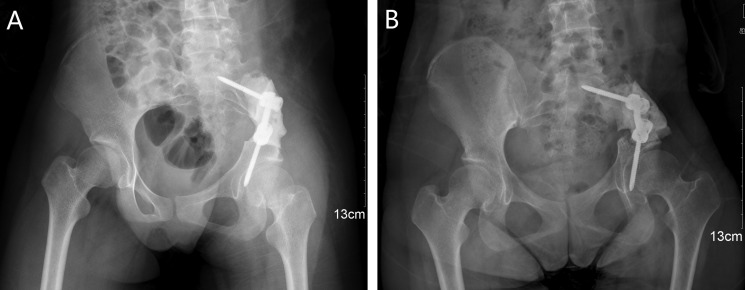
A 15-year-old female (case 28) with diagnosis of pelvis osteosarcoma affecting zone I (A) AP radiograph of the pelvis after bone tumor resection and reconstruction with screw-rod system and bone cement. (B) AP radiograph showing the implant loosening 14 months post-operatively without functional impairment.
